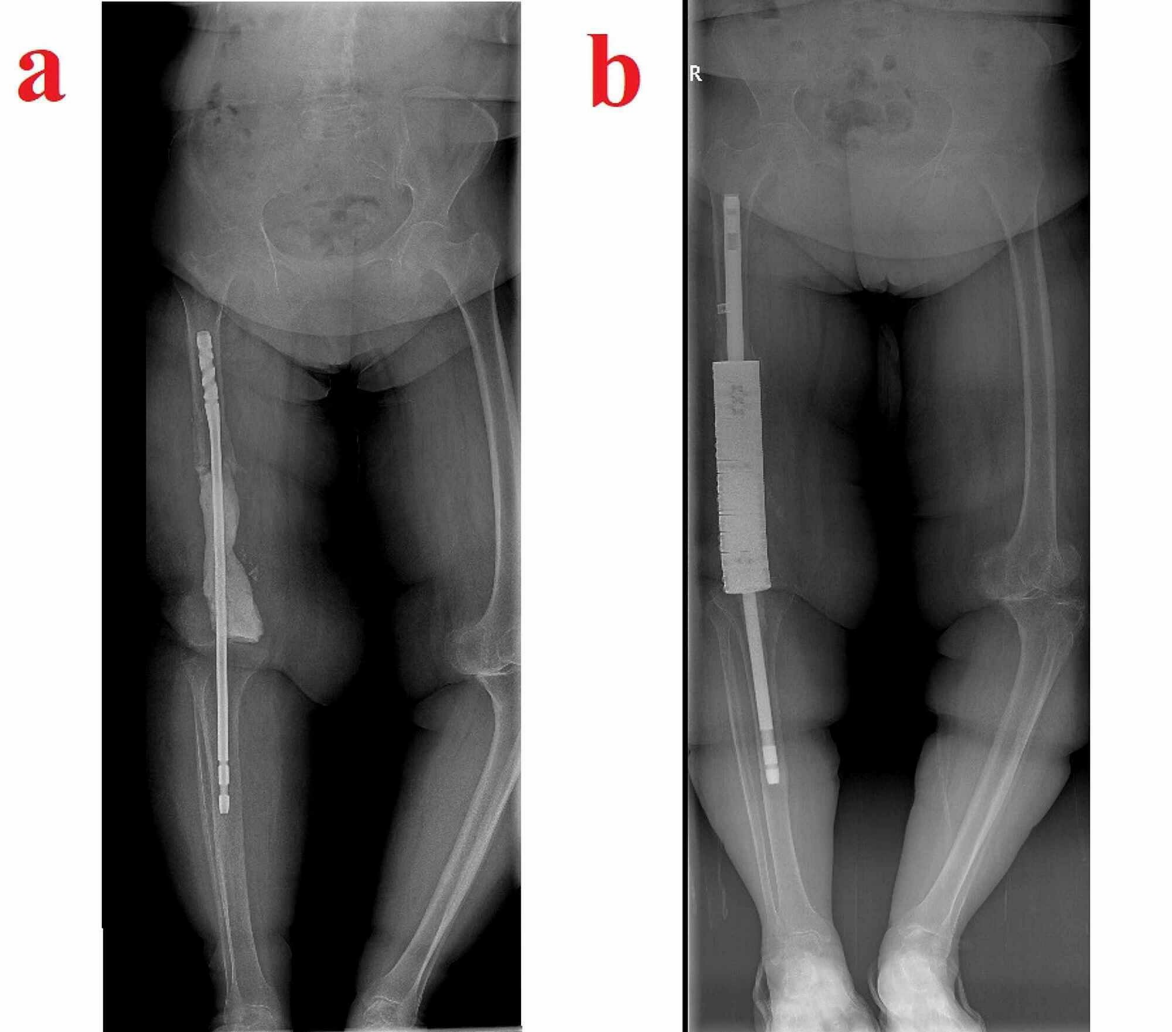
Images related to temporal arthrodesis and final standing radiograph The temporal arthrodesis performed after extensive segmental resection using a long knee-spanning femoral nail centrally covered with a PMMA block (a) and the final standing radiograph showing the segmental defect-bridging intramedullary knee arthrodesis system chosen for the case (b) PMMA: polymethyl methacrylate
